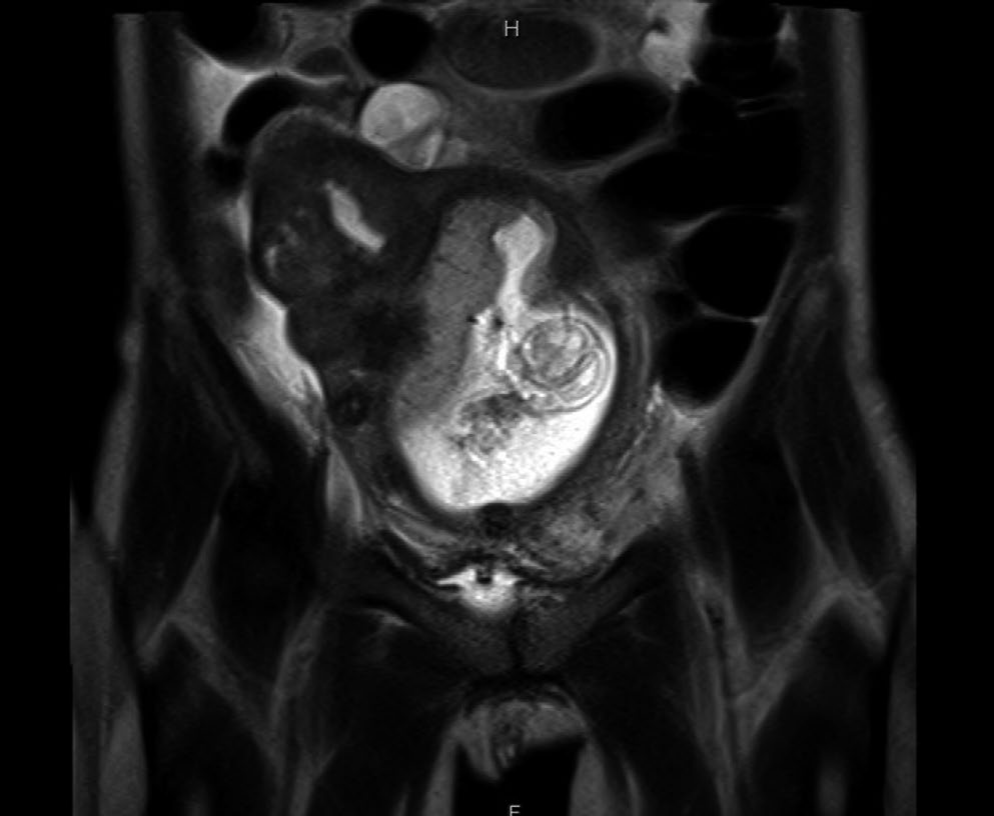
Coronal T2‐weighted magnetic resonance imaging showing a leiomyoma with partial high signal intensity at the uterine fundus, which was considered to be a degenerated leiomyoma
